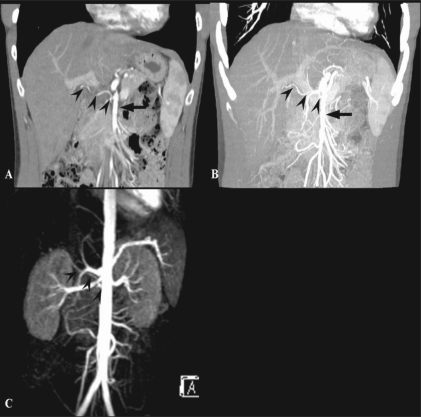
A 35-year-old man with a variation in HA anatomy. Arterial phase MPR (A) and MIP (B) CT scan images show the right HA (arrowheads) arising from the SMA (arrow). MRI (C) also demonstrates a variation in the right HA, Arterial phase MIP MRI also demonstrates the right HA (arrowheads) arising from the SMA (SMA is masked by the aorta). HA: hepatic artery, SMA: superior mesenteric artery
